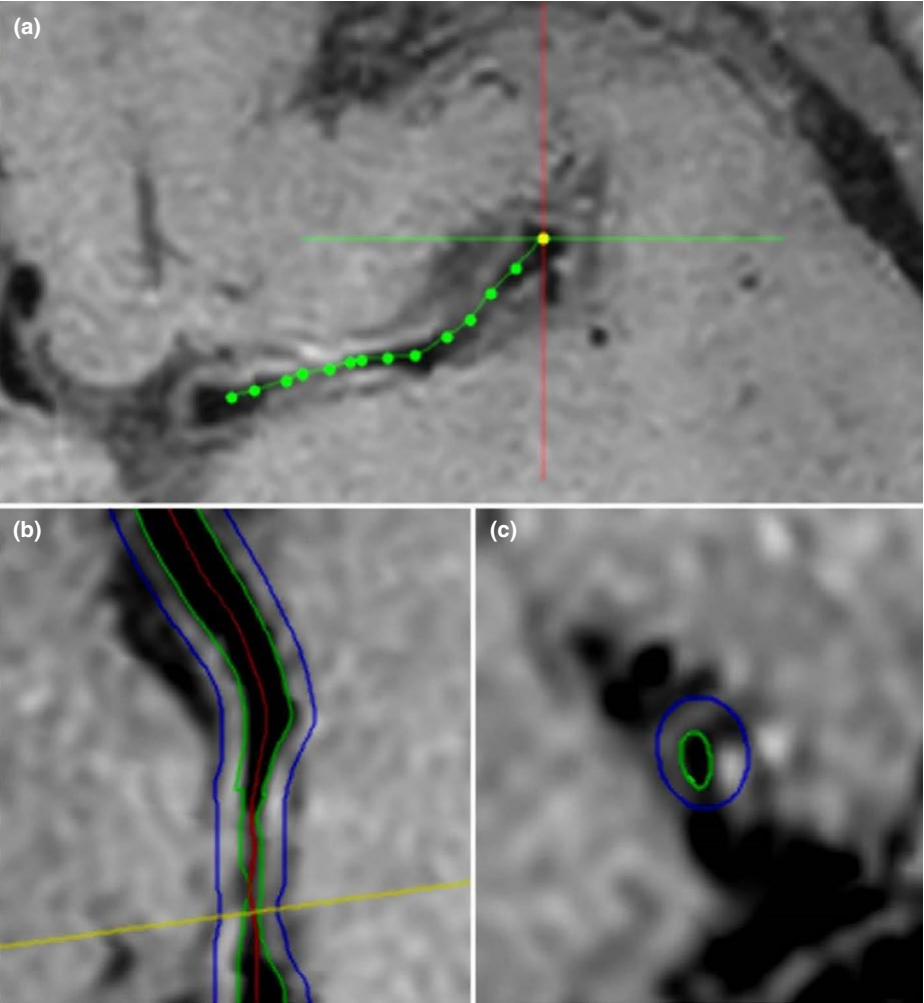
Work flow of the MR image analysis of intracranial artery using 3D‐CASCADE software. First step: outline of the center line of target segment of intracranial artery (a, M1 segment of middle cerebral artery); second step: automatic detection of the lumen and outer wall boundaries on the carved reconstructed image of target arterial segment (b); third step: manual adjustment of the lumen and outer wall boundaries on the axial image which is perpendicular to the center line (c)
